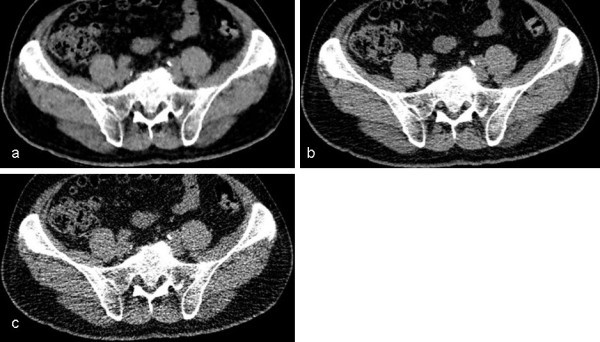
Noise and streak artifact of each CT image. Axial unenhanced CT images of UL-MBIR (a), L-ASIR (b), and UL-ASIR (c) of a 61-year-old man weighing 75 kg. Less noise and fewer streak artifacts are seen in UL-MBIR than in L-ASIR and UL-ASIR.
